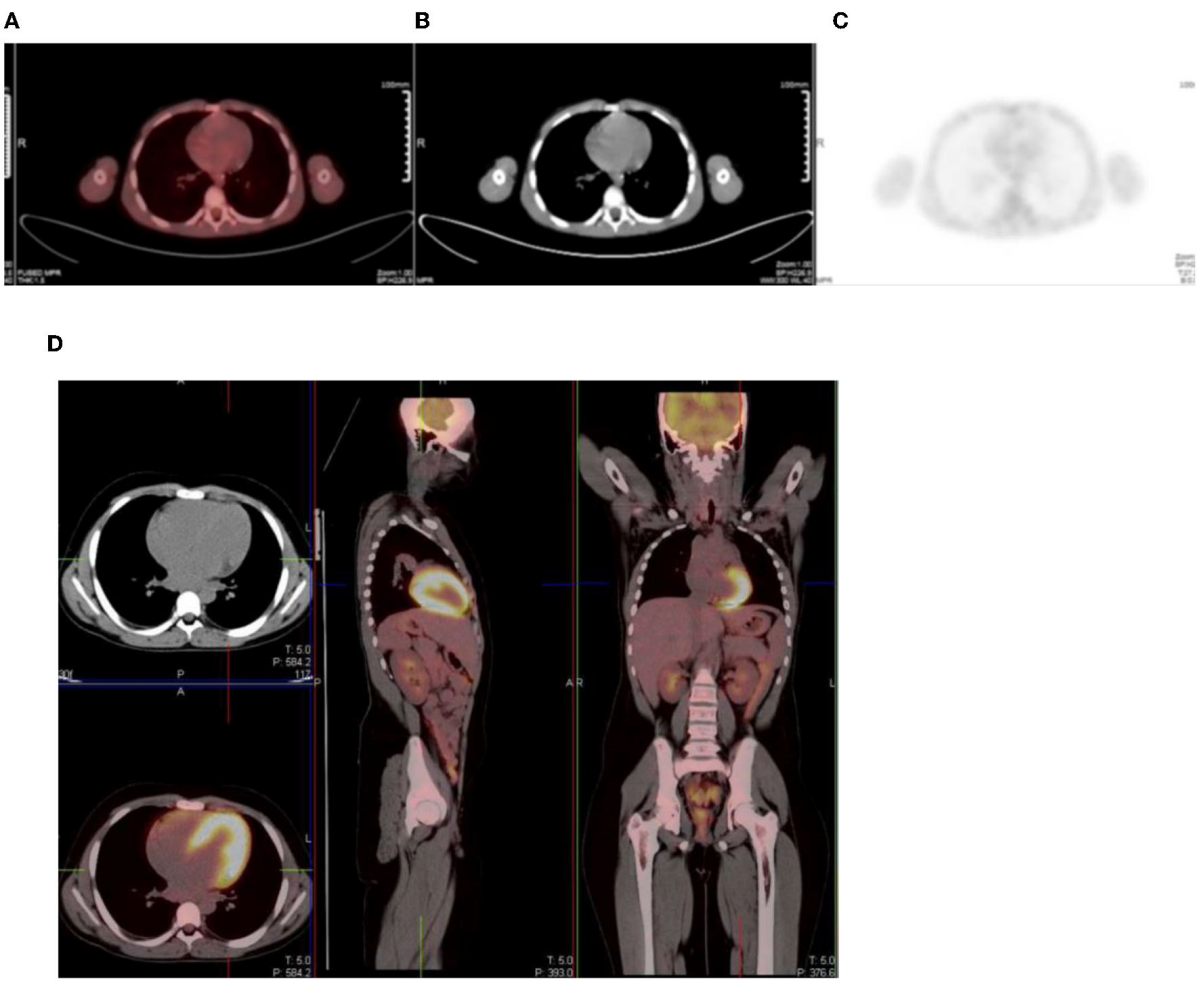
(A–C) Axial fused FDG PET/CT (A), CT (B) and PET (C) images showing a 16 year old male who was well fasted and shows no/minimal cardiac uptake. (D) Transverse, sagittal and coronal PET/CT in a 13 year old boy with intense physiological FDG uptake in the left ventricle of the myocardium.
